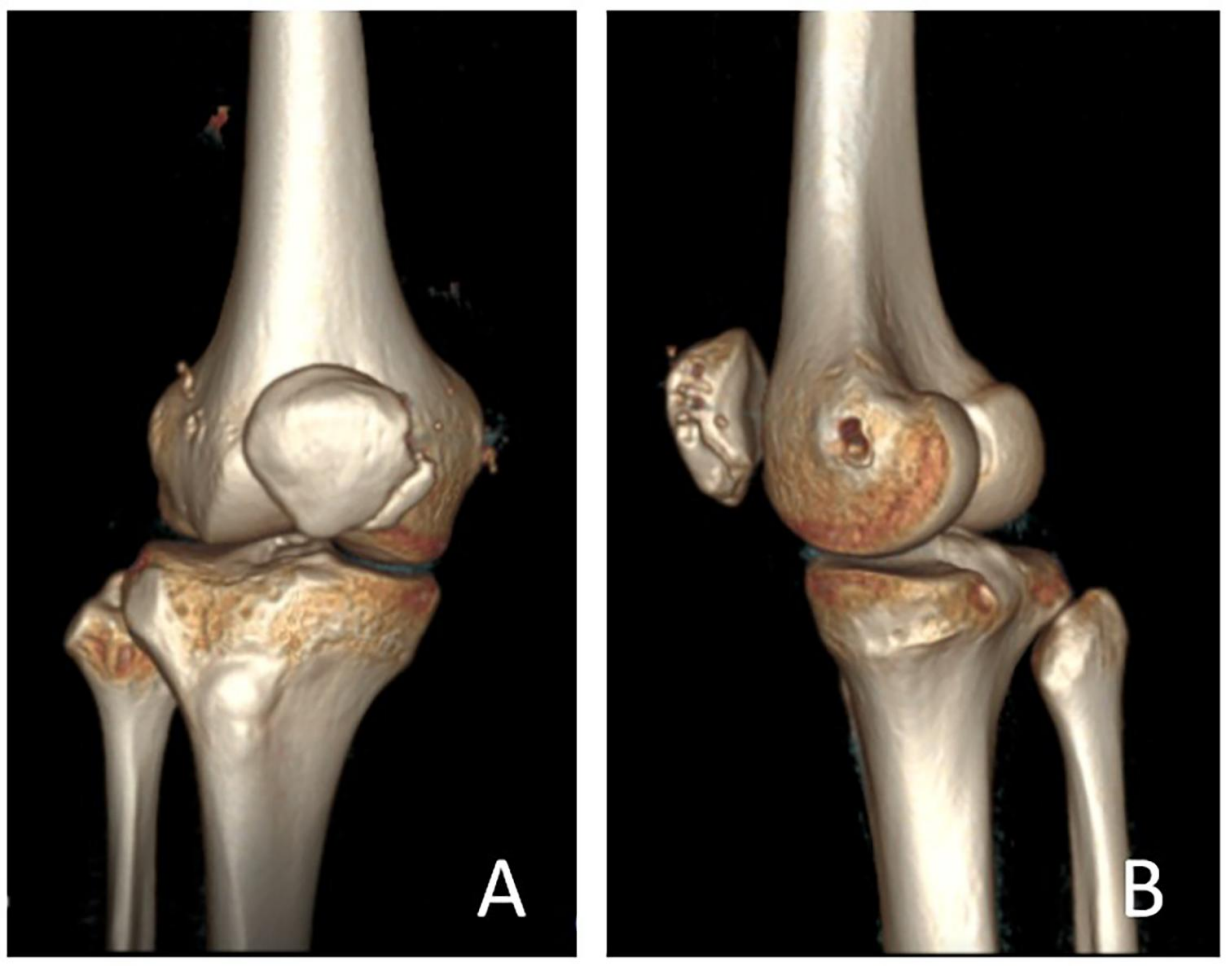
Six-month postoperative right knee CT scan with 3D reconstruction. Follow-up CT imaging and three-dimensional reconstruction at six months postoperatively demonstrate the right patella in normal anatomical position, indicating restoration of patellar alignment. (A) Frontal view. (B) Lateral view.
